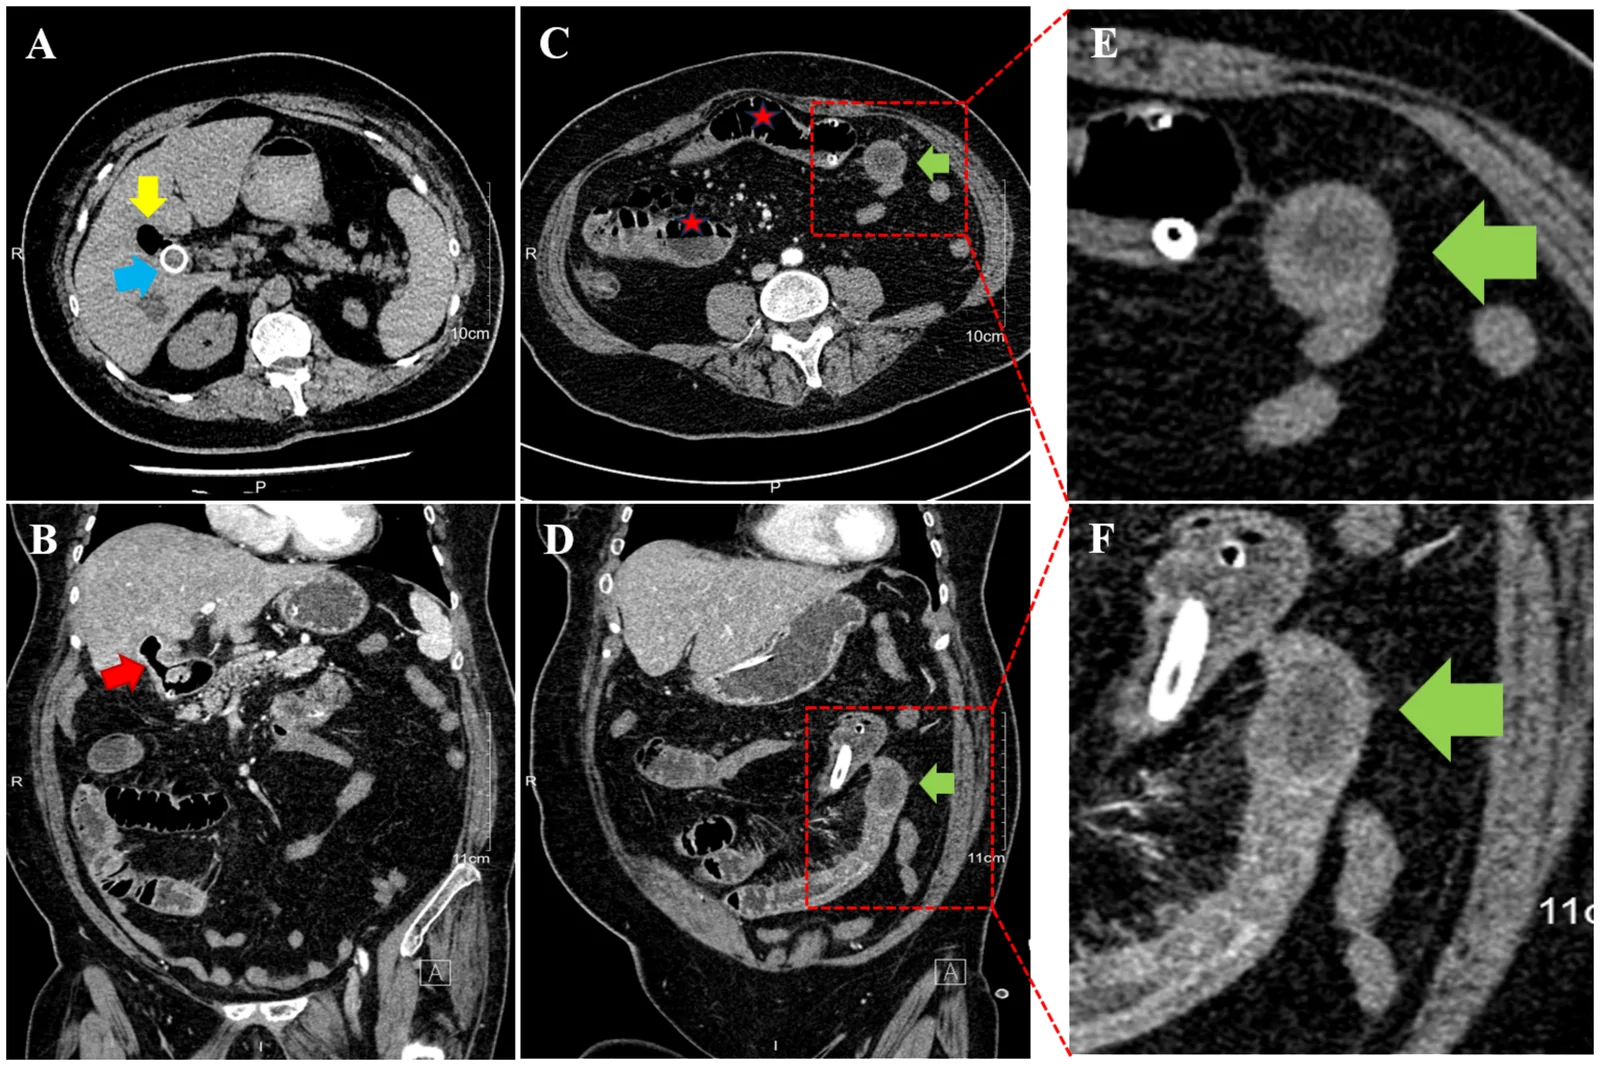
Abdominal CT scan in coronal and axial planes. (A) Pneumobilia with gas in the gallbladder (yellow arrow) and a gallstone with rim calcification (blue arrow). (B) Internal fistula between the gallbladder and duodenum (red arrow). (C, D) Contrast-enhanced CT showing a faintly rim-calcified gallstone within the jejunum (green arrow) and air-fluid levels in the bowel (pentagram). (E, F) Magnified images of the jejunal stone (green arrow).
